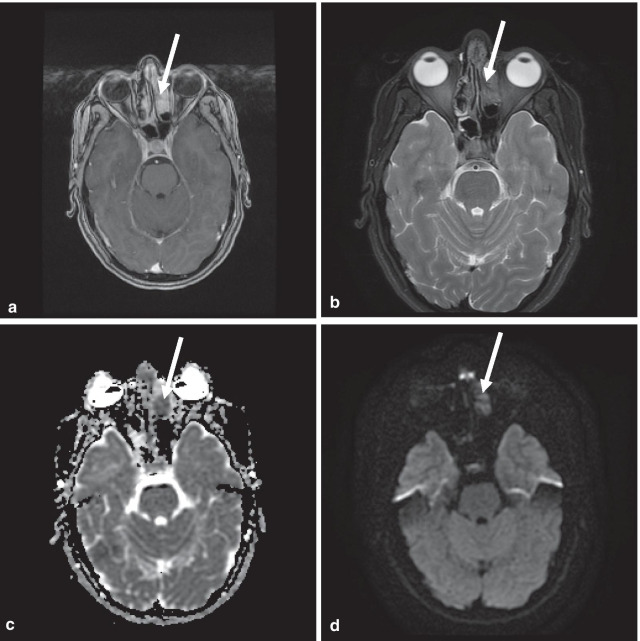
Contrast-enhanced maxillofacial MRI demonstrates left ethmoid sinus lesion. a Enhancing infiltrative lesion in the left anterior ethmoidal sinus in the Gadolinium-enhanced sequence (White arrow). b This lesion is hypointense in the T2-weighted sequence. c, d The lesion is hypointense in the apparent diffusion coefficient (ADC) sequence and mildly hyperintense in the diffusion-weighted imaging (DWI) sequence, likely due to hypercellularity
